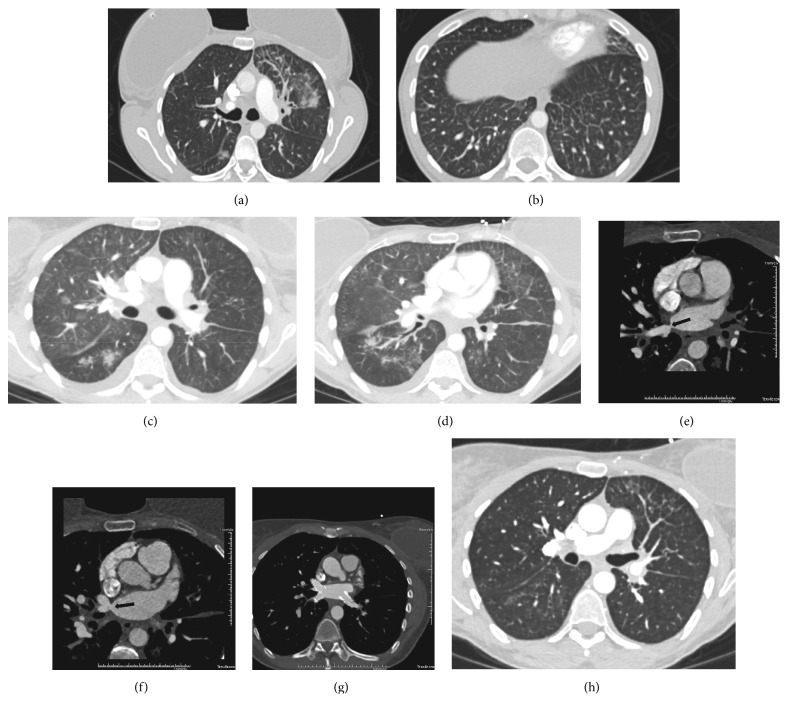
(a) and (b) Axial computed tomography (CT) images of prepulmonary vein stenting demonstrate patchy opacities and ground-glass and septal thickening reflecting manifestations of pulmonary vein stenosis with congestion and edema. Opacities are most pronounced in the left upper lobe secondary to complete occlusion of the superior left pulmonary vein ostium. (c) and (d) Follow-up axial CT images of prepulmonary vein stenting demonstrate progression of patchy opacities and ground-glass compatible with increased pulmonary venous congestion and edema. Additionally, small pleural effusions are now visible. (e) and (f) Cardiac CT shows narrowing of the right superior and inferior pulmonary vein ostia (arrows). Although not shown, narrowing of the left inferior ostium and complete occlusion of the left superior pulmonary vein ostium was noted. (g) Contrast-enhanced CT of postpulmonary vein stenting demonstrates marked improvement of pulmonary vein narrowing. The superior left pulmonary vein ostium was not stented and remained occluded. (h) Axial chest CT in lung windows shows substantial improvement of ground-glass and septal thickening. Findings remain the most pronounced in the left upper lobe, as the superior left pulmonary vein ostium was not stented.
